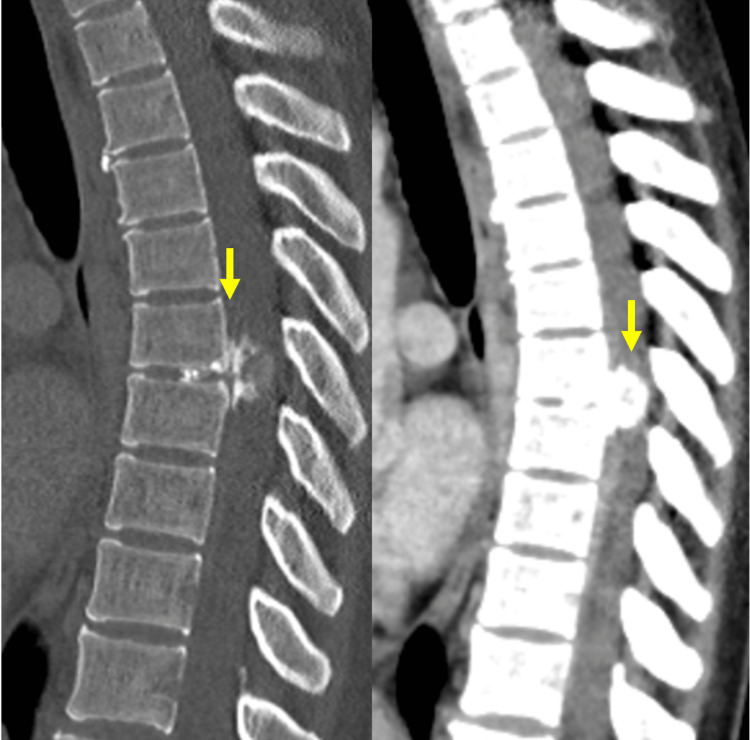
CT scan showing calcified lesion in T7 level. Arrow: calcified lesion.
